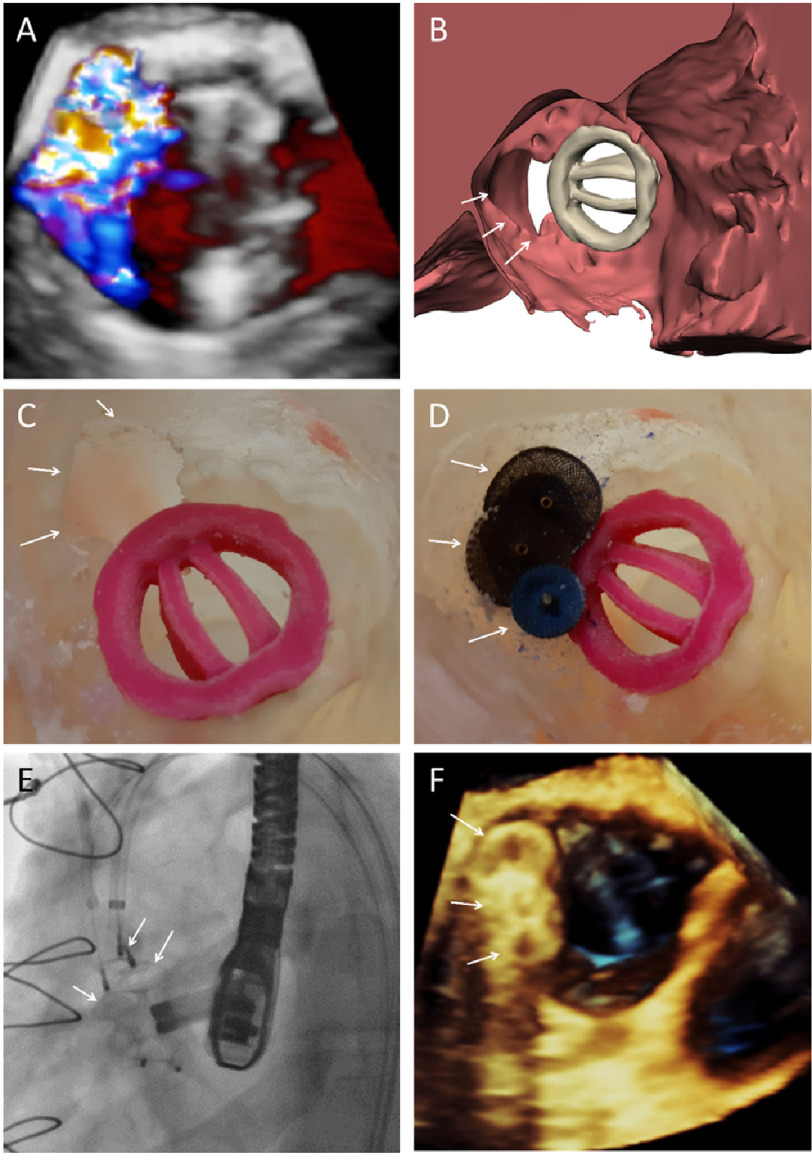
The first case. A. 3D TEE showing the defect at 8–11 o’clock. B. CT segmentation showing the defect. C. 3D model of the defect. D. Bench test using three AVP II. E. Percutaneous closure using planned plugs. F. 3D TEE showing well seated plugs.
